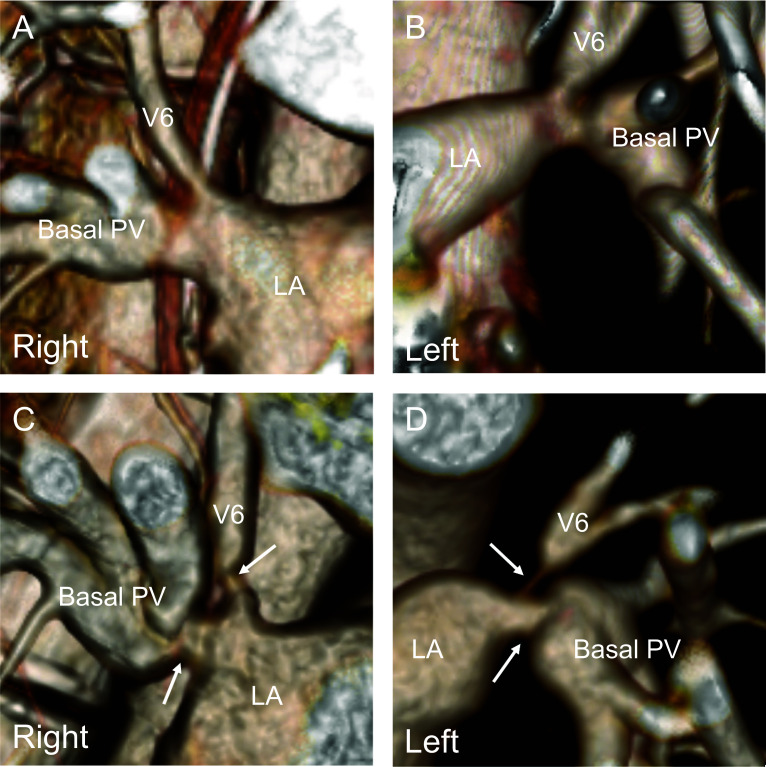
Three-dimensional computed tomographic angiography (A and B 5 days after living lobar lung transplantation, C and D 6 months after living lobar lung transplantation) showing progressive bilateral pulmonary vein obstructions (arrows). PV: pulmonary vein, LA: left atrium
